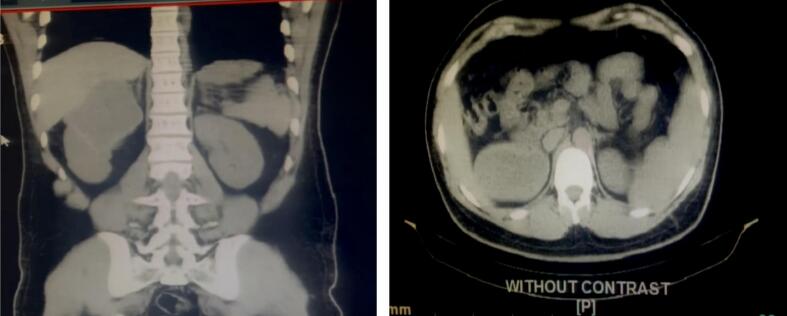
Abdominal CT scan demonstrating a heterogeneous cystic lesion with internal septations and echogenic foci, consistent with a stage 4 chronic hydatid cyst.
